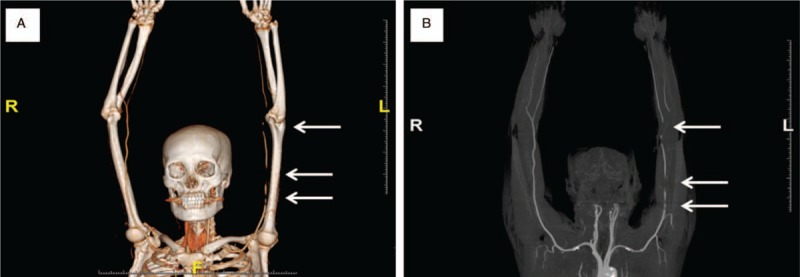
CT angiography 3-dimensional image findings showed multifocal total occlusion of left brachial artery (white arrows) with reconstitution at proximal radial and ulnar arteries suggesting acute thrombosis (A, B).
